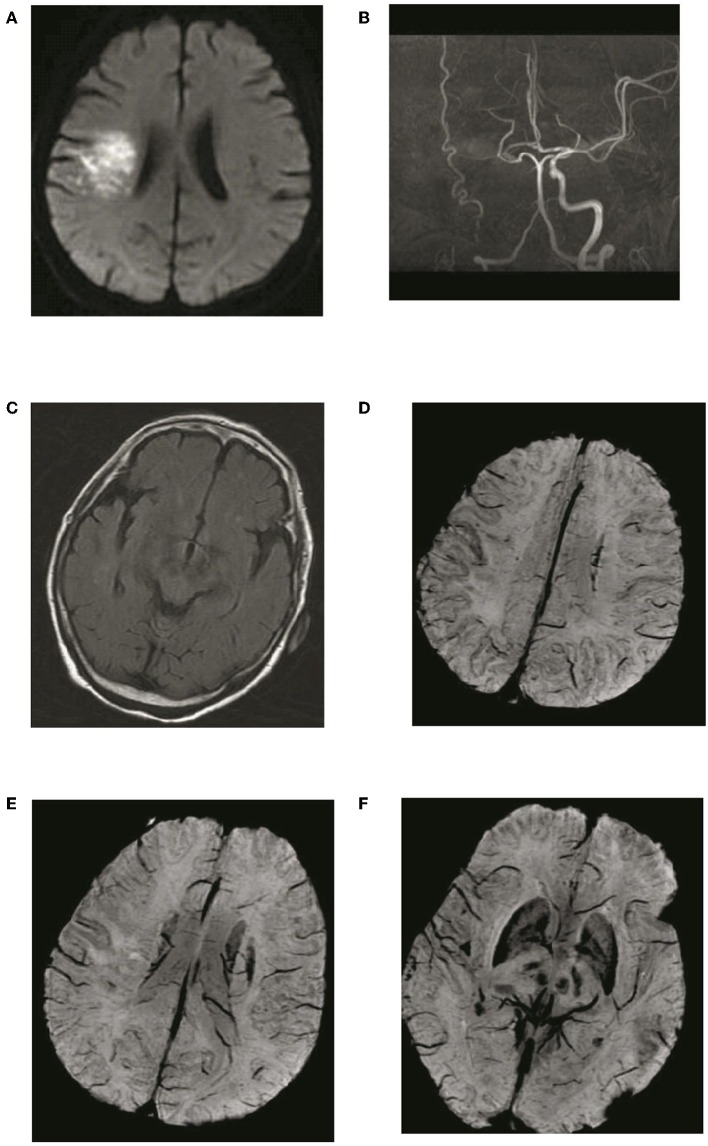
Typical patient with absence of asymmetric deep medullary veins (ADMV). A 69-year-old female presented with left limb weakness for 5 h. (A) The right parietal lobe shows acute corona radiata cerebral infarction on DWI; (B) Occlusion of the right internal carotid artery on magnetic resonance angiography with maximal intensity projection; (C) HVS with a score of 0 on T2-FLAIR image: (D) Absence of ADMV; (E,F) ACV with a score of 2 on SWI.
